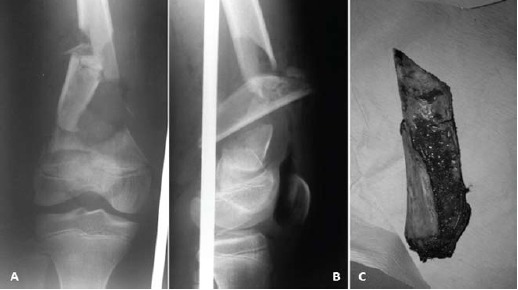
Fig. 2: Radiographs at 1 year showing incorporation of the graft with fracture union F2:
